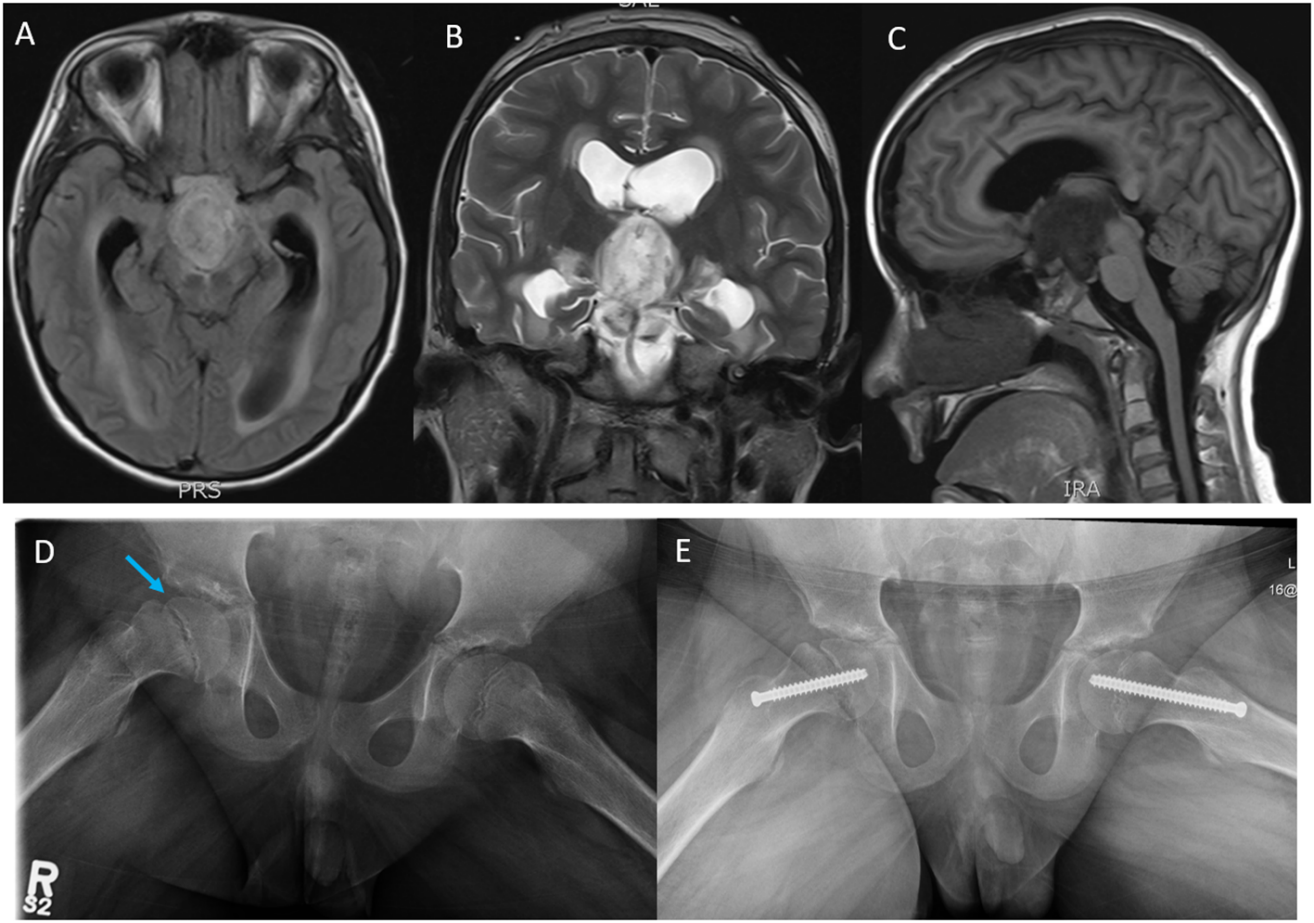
Magnetic resonance imaging (MRI); (A) axial T1 flair, (B) coronal T2, and (C) sagittal T1 flair images showing a rim-enhancing tumor mass likely arising from the Optic chiasm and extending into the third ventricle, the prepontine cistern, the interpeduncular cistern intermittent to the optic chiasm measuring 3.0 cm (AP) × 2.7 cm (CC) ×1.8 cm (T). (D) Plain X-ray films, open frog leg view showing inferior displacement of the right femoral capital epiphysis (blue arrow) relative to the femoral neck associated with widening of the proximal femoral growth plate and minimal joint effusion. (E) Surgical pinning to stabilize the capital epiphysis on the right hip and prophylactic pinning to prevent SCFE on the left hip.
